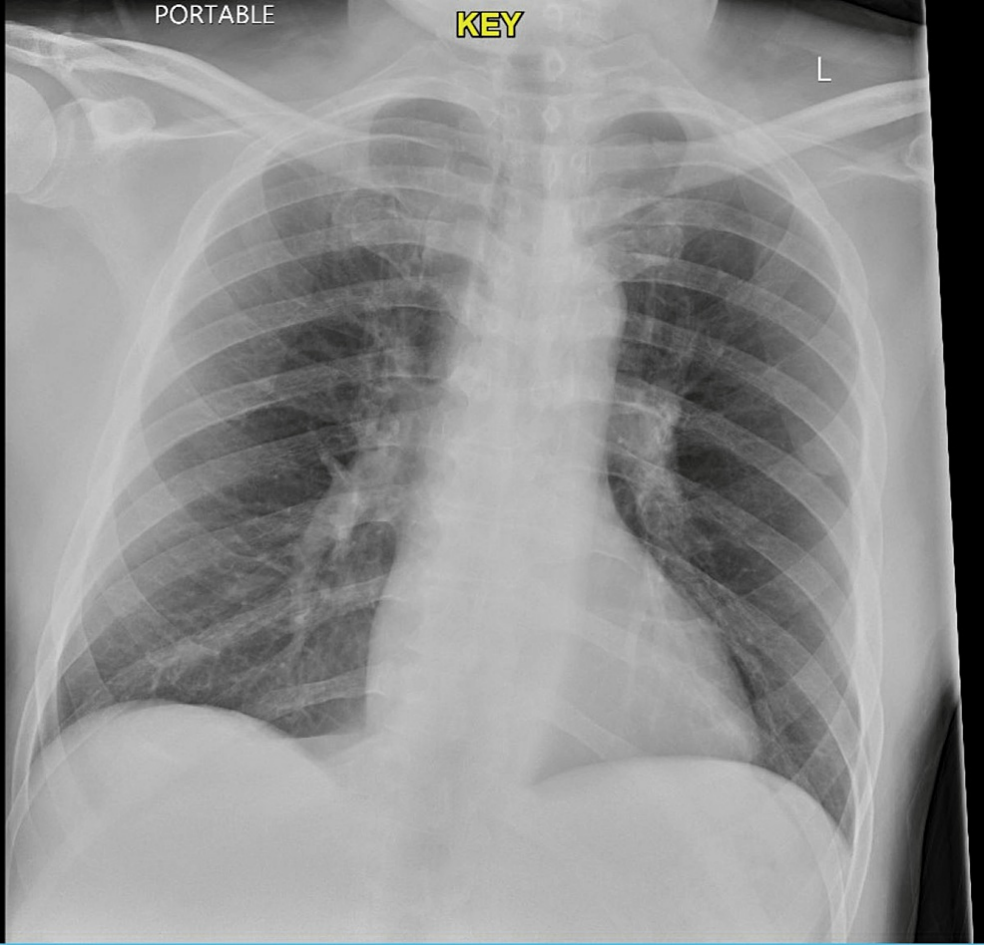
Chest x-ray at the time of admission.
